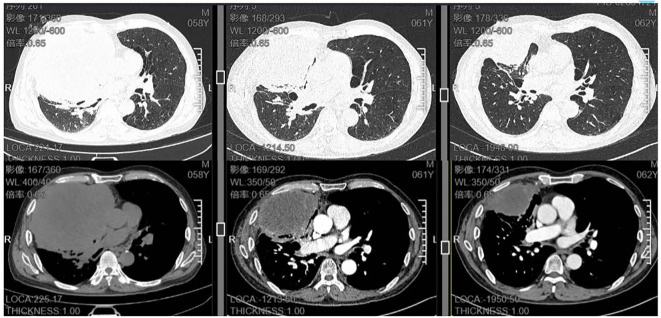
Chest computed tomography (CT) images during treatment. Representative CT images obtained before chemoimmunotherapy (July 2023, left), after 6 cycles of chemoimmunotherapy (November 2023, middle), and after consolidative thoracic radiotherapy (January 2024, right), demonstrating marked regression of the primary lung tumor over the course of treatment.
